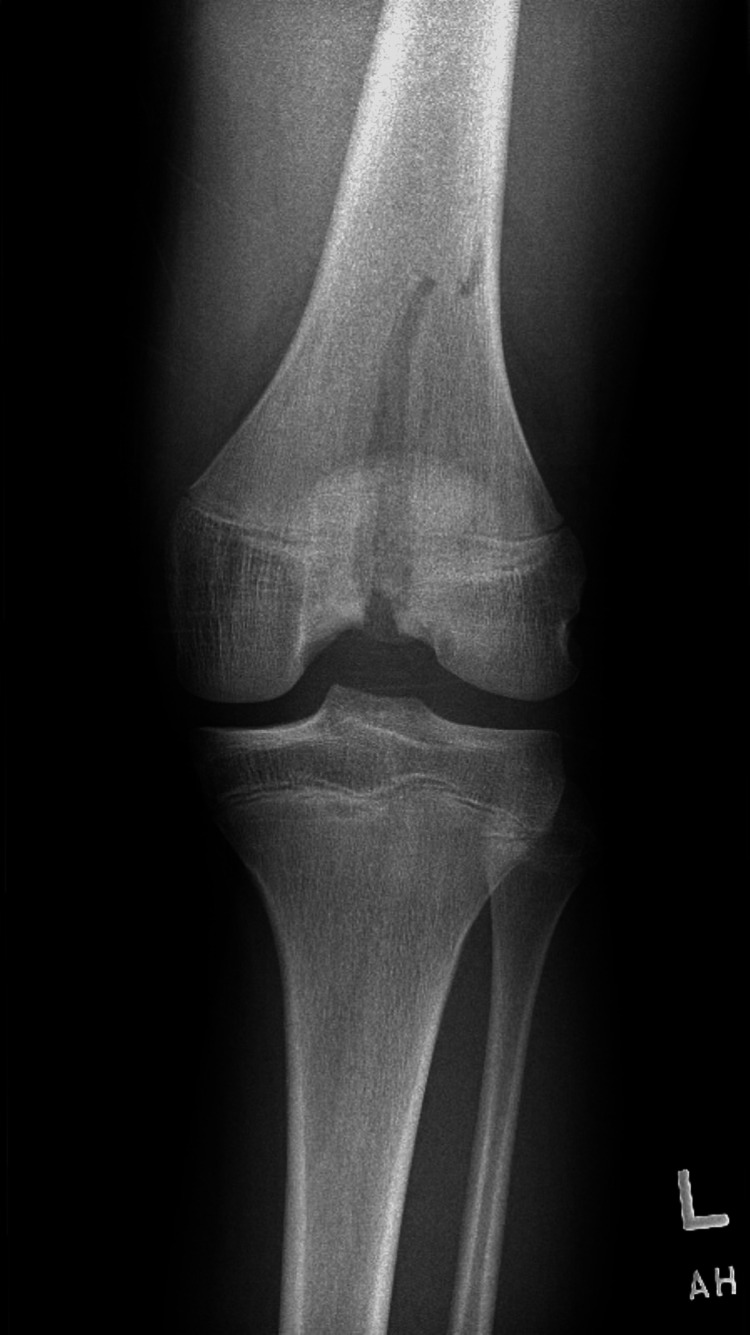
Left knee radiograph, anteroposterior view
